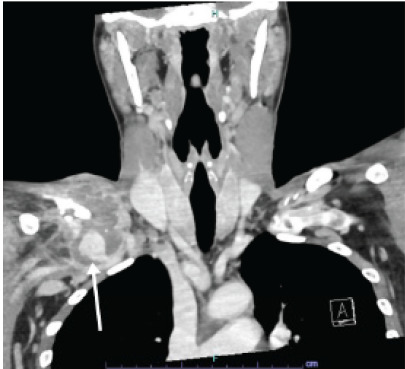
Computed tomography with contrast coronal view of right subclavian artery pseudoaneurysm (arrow).
